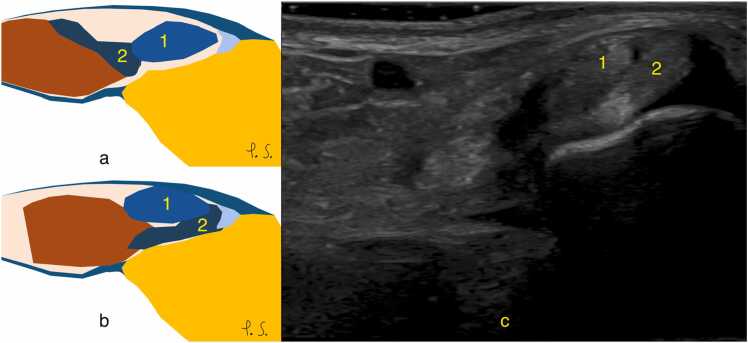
Instability of the peroneal tendons without a split or superior peroneal retinaculum tear. Normal anatomy is shown on a. Intra-sheath luxation (b and c): the peroneus brevis (2) is completely positioned under the peroneus longus (1) and is displaced laterally. Transverse cross-section (c) at the level of the lateral malleolus, ultrasound performed during dorsiflexion and eversion.
